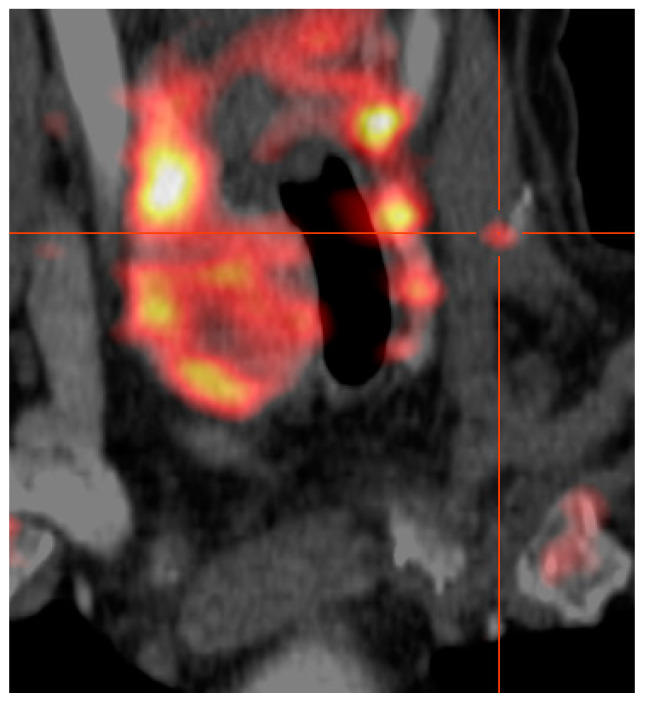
Autotransplanted tissue of a parathyroid adenoma, 18 years after initial surgery depicted by FEC-PET-CT-scan and localized into the left sternocleidoid muscle next to a clip. To the right of the trachea, the right thyroid lobe is seen and to the left of the trachea a chain of parathyroid adenomata, caused by parathyreomatosis is noted.
